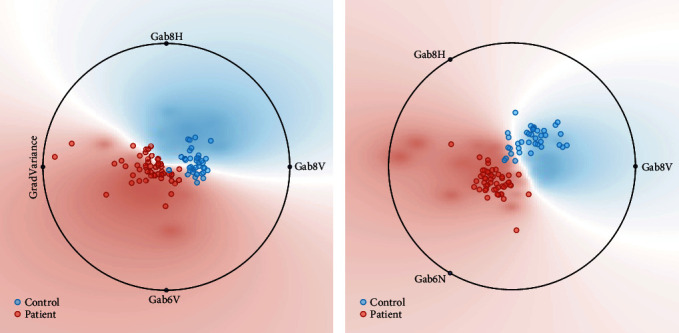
Feature space of the best radiomics feature combinations of R-wave ultrasound images and T-wave ultrasound images. (a) Random forest and (b) Support Vector Machine classifiers.
